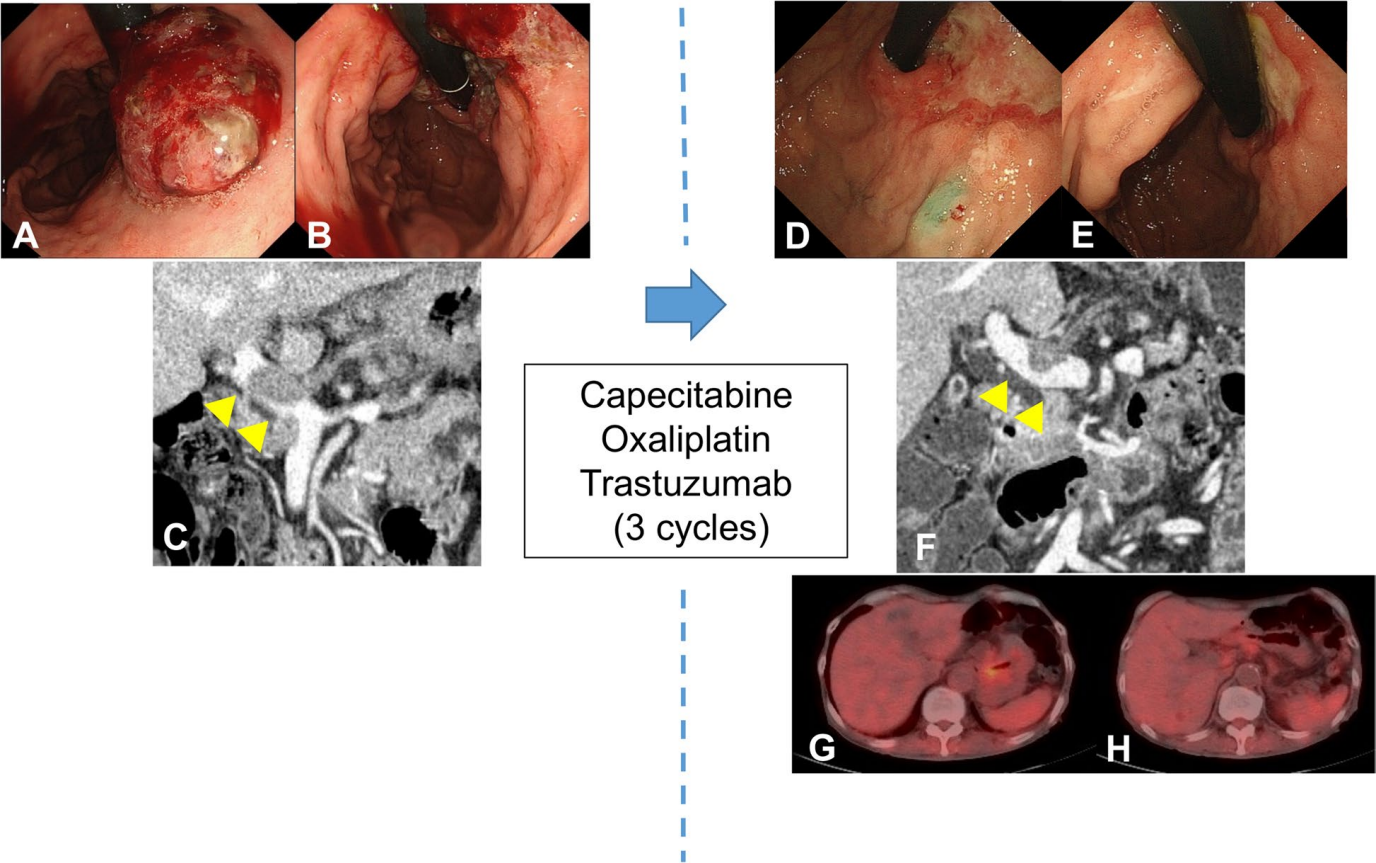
Gastrointestinal endoscopy revealed an elevated tumor on the side of the lesser curvature, from the middle of the gastric body to the cardia. The length of the tumor was approximately 5 cm before chemotherapy (A, B). Computed tomography revealed portal vein tumor thrombus (PVTT) (C). After chemotherapy, the primary gastric lesion flattened and the PVTT shrank (D–F). Arrowheads show PVTT (C, F). Positron emission tomography only showed abnormal uptake in the primary gastric lesion and PVTT (G, H)
